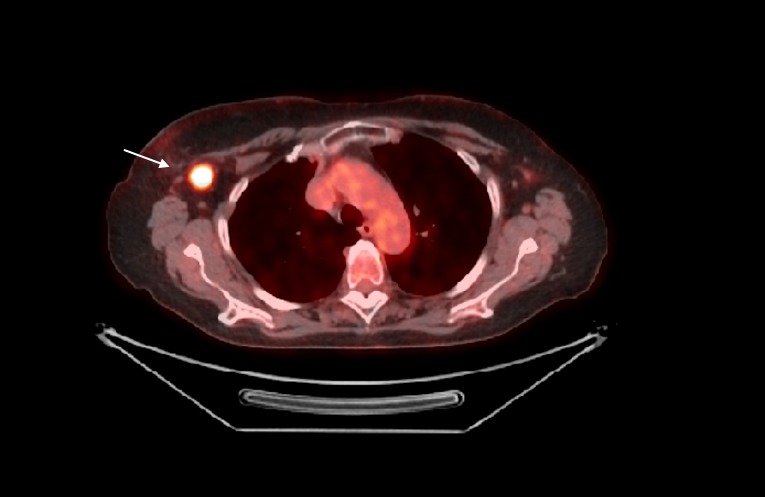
PET/CT scan shows a contralateral recurrence of the angiosarcoma (white arrow) PET/CT: positron emission tomography/computed tomography
